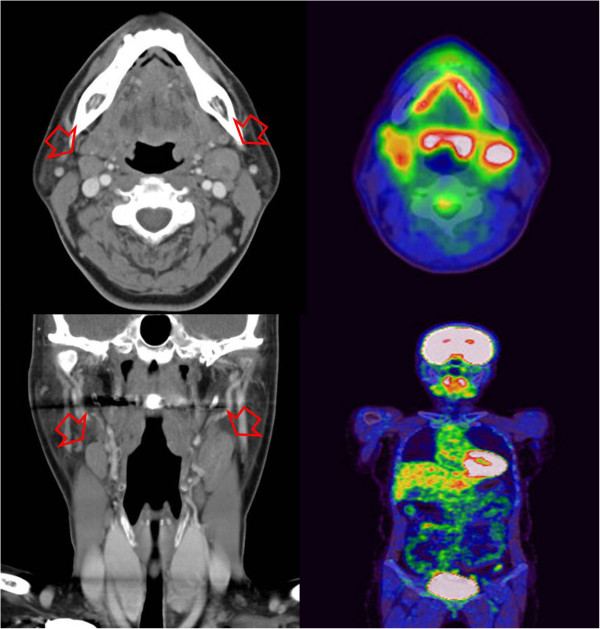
Neck CT and whole-body PET-CT obtained 27 months after lobectomy. The neck CT shows that the lymph nodes on both sides of the neck are enlarged (arrows), and the PET-CT discloses increased 18F-FDG uptake in the mid-portion of the tongue base, and enlarged lymph nodes. 18F-FDG, 18F-fluorodeoxyglucose; CT, computed tomography; PET, positron emission tomography.
